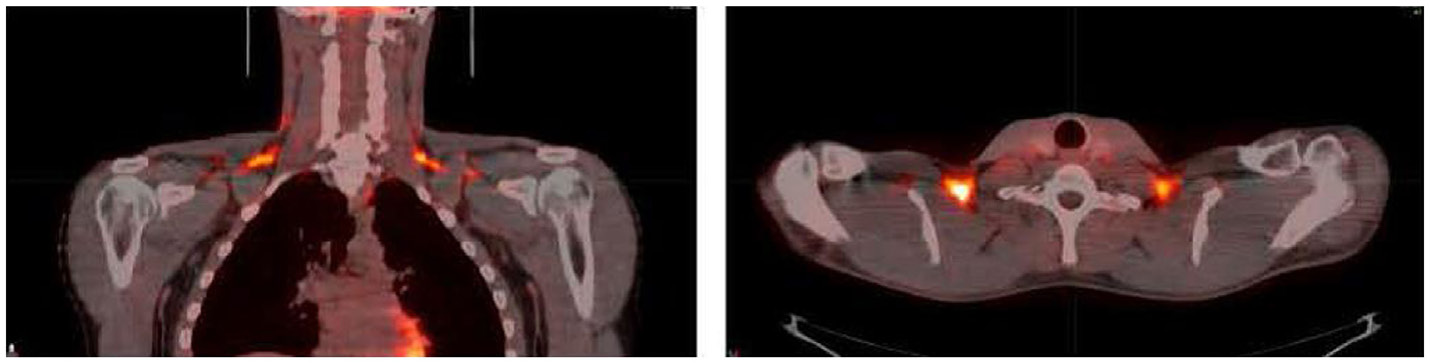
Coronal (left) and transverse (right) 2-deoxy-2-[18F]fluoro-d-glucose (^18^F-FDG) positron emission tomography (PET)-computed tomography (CT) images from a volunteer during cold exposure. The intense orange color in the supraclavicular area corresponds to brown adipose tissue. Image from Ref. (24). Reprinted with permission.
